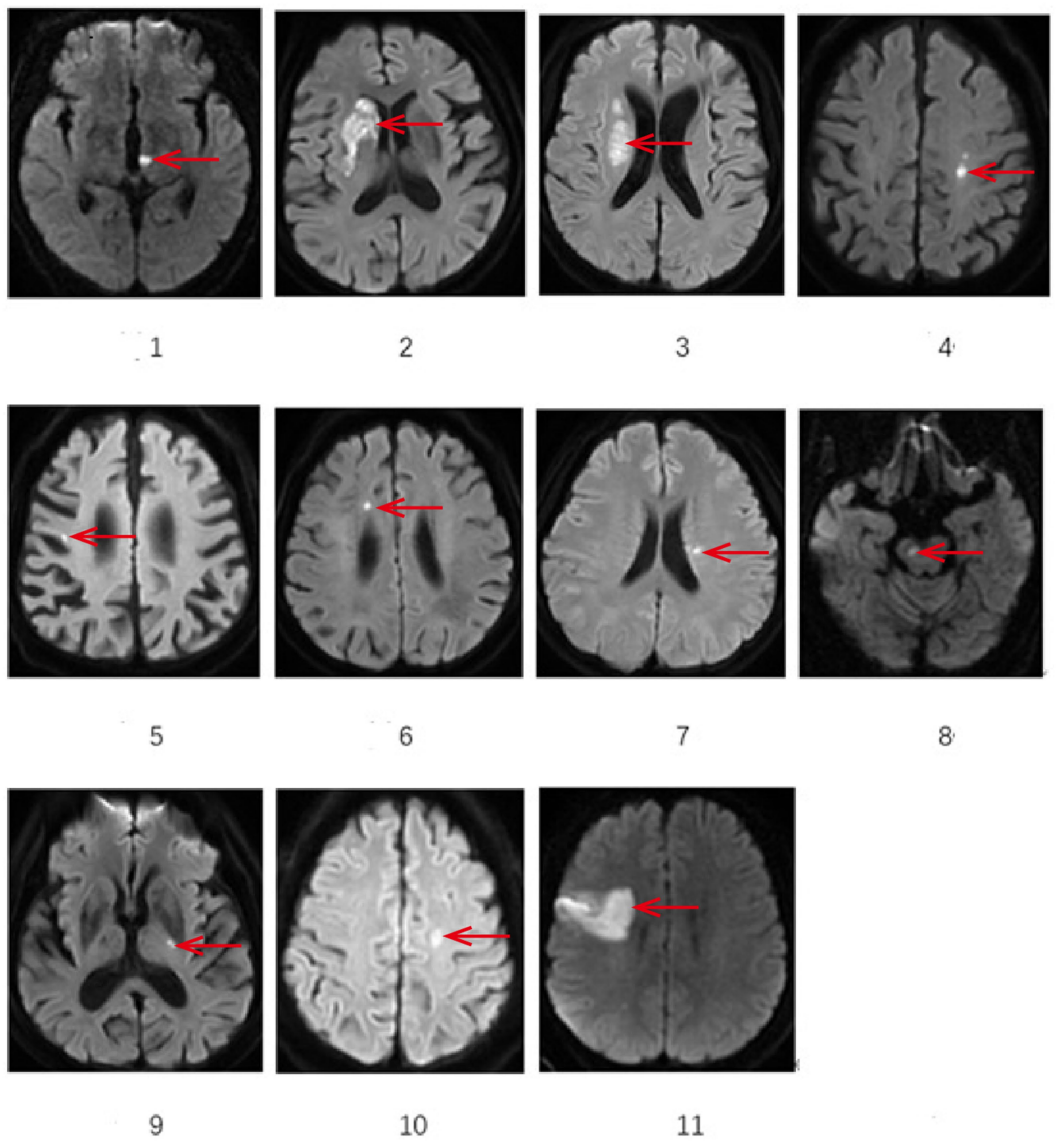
Demonstrates the representative sections of DWI sequences showing infarction sites on cranial MRI. *The area indicated by the arrow: 1 Left thalamus 2 Right basal ganglia 3 lateral ventricle periphery 4 left centrum semiovale 5 Right frontal lobe 6 Right frontal lobe 7 lateral ventricle periphery 8 Right cerebral peduncle of midbrain 9 Left basal gangli 10 Left centrum semiovale 11 Right frontal lobe.
